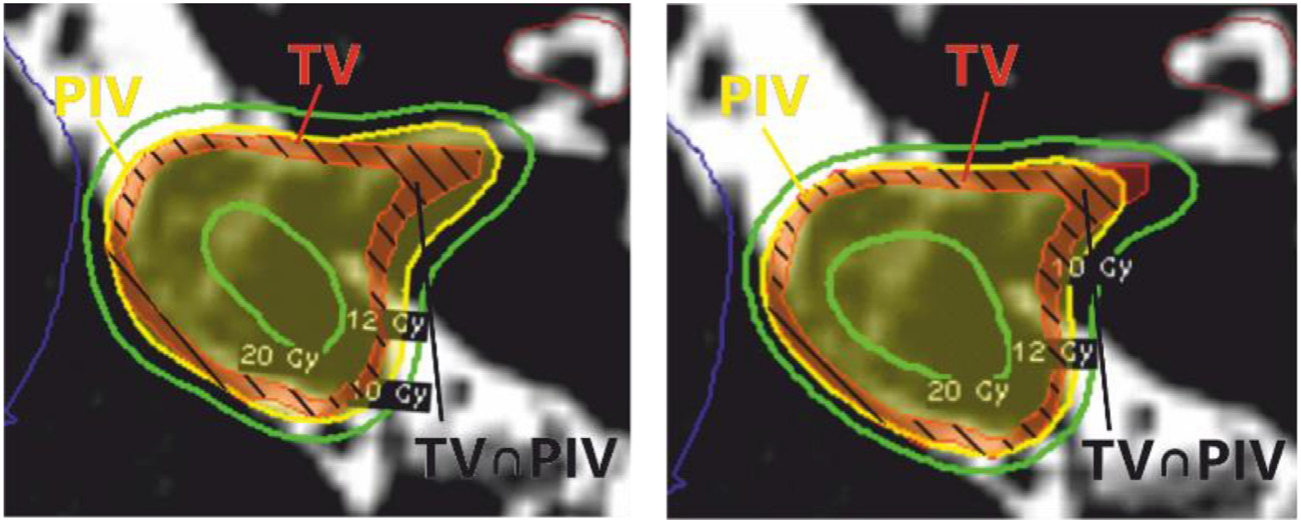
Horizontal T2-weighted magnetic resonance imaging scans demonstrating the comparison between the inverse planning strategy and Leksell GammaPlan for a representative acoustic neuroma case adjacent to the brain stem. Left, Leksell GammaPlan with *C* = 0.99 and *S* = 0.84. Right, inverse planning with *C* = 0.98 and *S* = 0.94. The red-black shaded area represents the intersection of the TV (red) and the 12-Gy prescription isodose (yellow).
